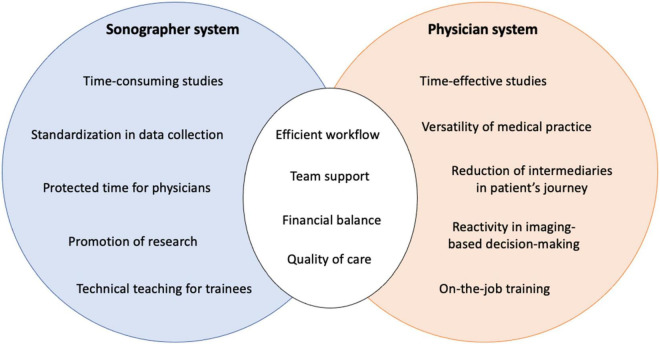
Strengths, weaknesses and common objectives for the sonographer and the physician systems.
